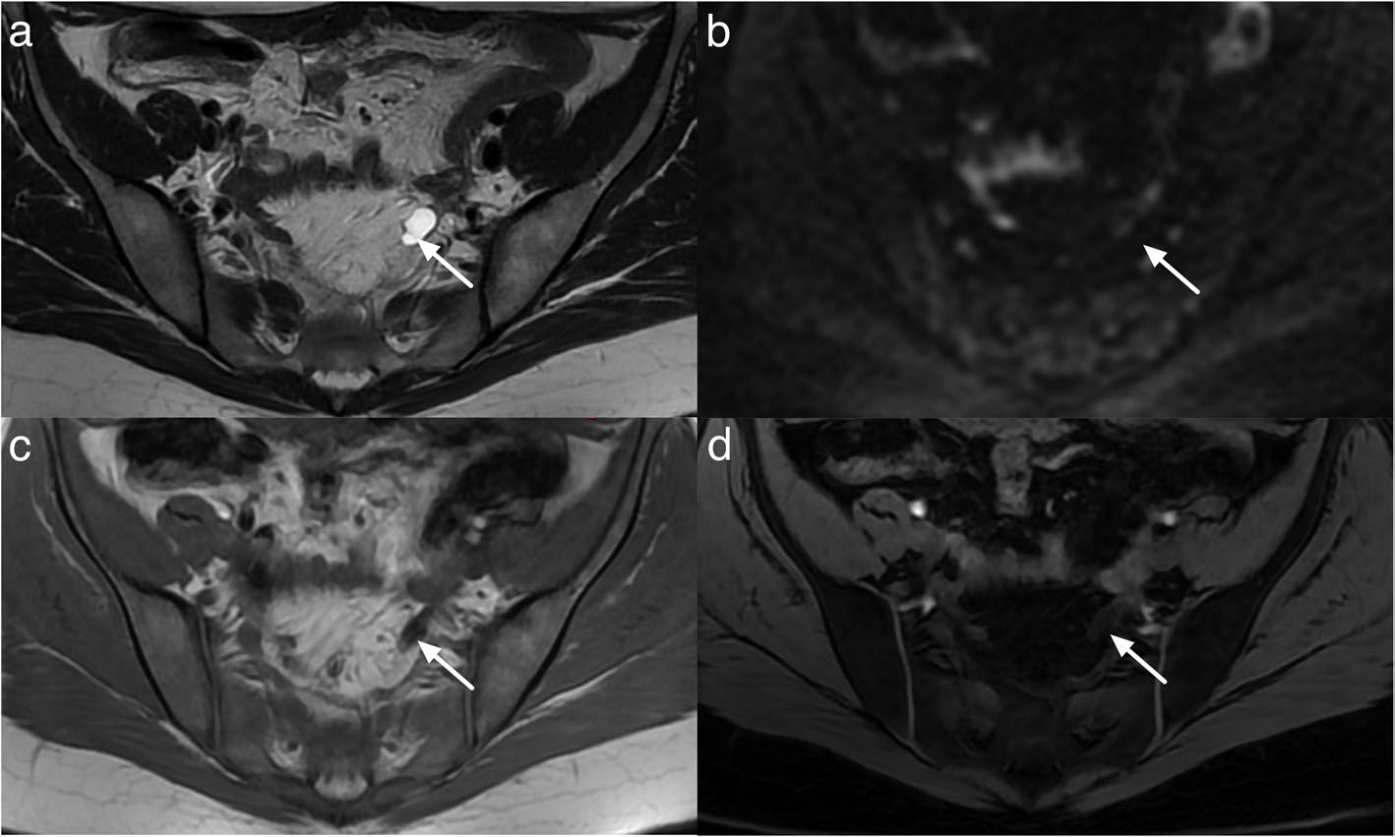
Example of MRI appearance of a para-ovarian cyst. a Axial T2-weighted sequence. b Axial DWI lombo-pelvic scan (*b*: 1200 s/mm^2^). c Axial unenhanced T1-weighted in-phase sequence. d Axial late-gadolinium T1-weighted sequence. Left para-ovarian cyst (maximal diameter: 17 mm) white arrow
